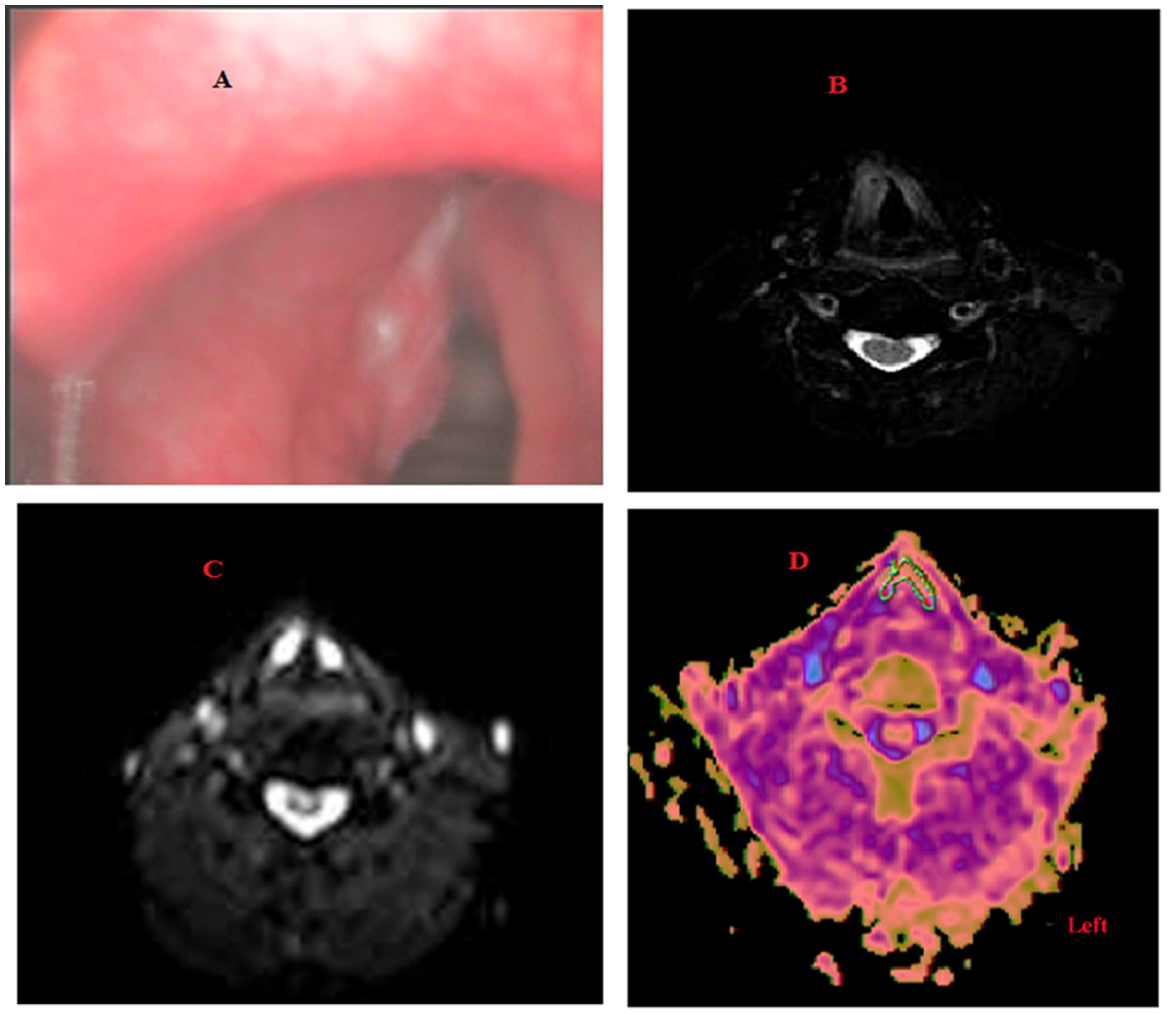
A patient with pathologically proven T_1b_N_0_M_0_ laryngeal carcinoma. A: A lesion was only found in the right vocal cord, and another lesion in the left vocal cord was not found by laryngostroboscopy. B: Transverse T2-weighted MRI showed a tumor in the right vocal cord. C: DWI suggested lesions were in the bilateral vocal cords (b = 1000 s/mm^2^). D: Consequently, the corresponding ADC map revealed hypointense lesions in the bilateral cord (ADC = 1.05×10^−3^ mm^2^/s in the left vocal cord, ADC = 0.90×10^−3^ mm^2^/s in the right vocal cord).
